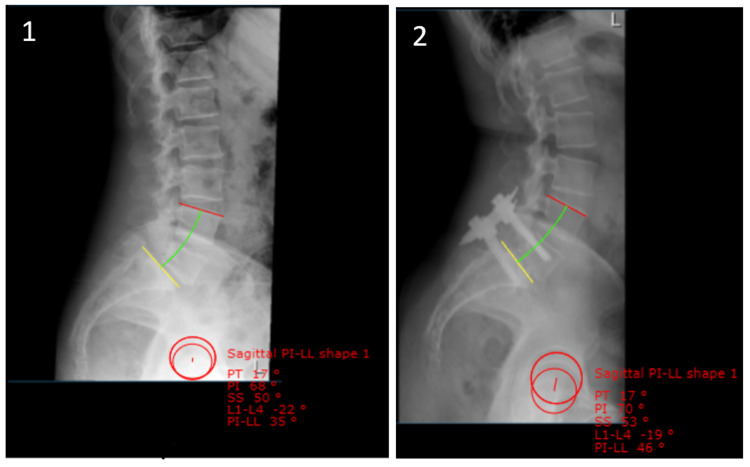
Pre-operative (1) and most recent post-operative (2) standing lateral pelvic radiographs.
